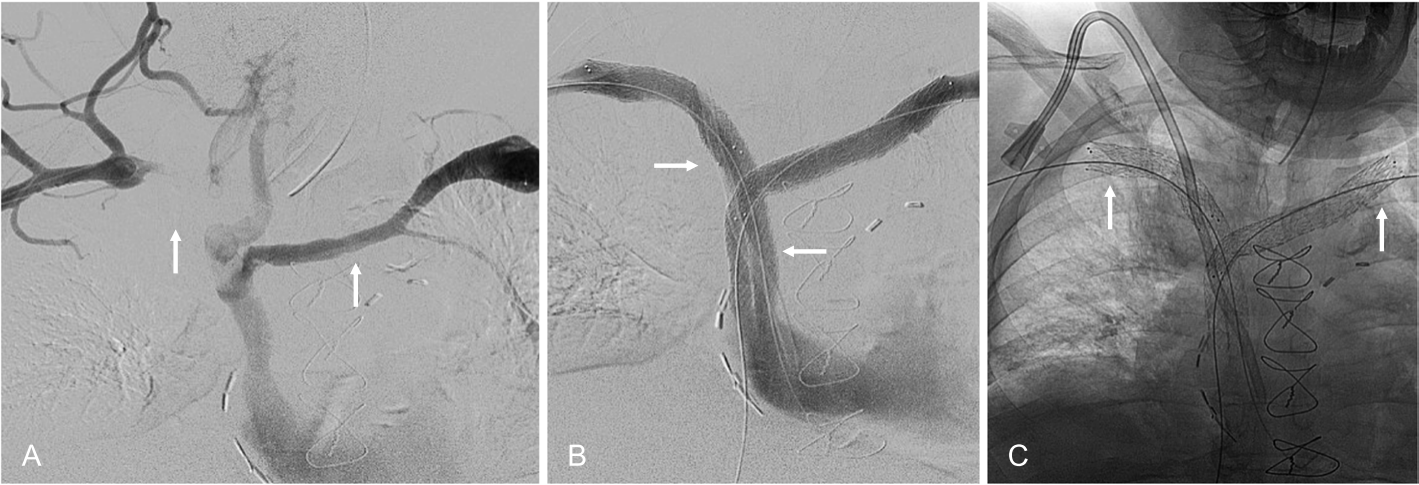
65-year-old male with end-stage renal disease with superior vena cava syndrome* (A) Bilateral upper extremity venography demonstrating bilateral brachiocephalocaval vein occlusion (arrows) (B) Bilateral upper extremity venography, after bilateral brachiocephalocaval stent reconstruction using bilateral 11-mm VBX balloon expandable endoprostheses (centrally) and 14-mm Abre Venous Stents (peripherally) (arrows), showing brisk in-line from both upper extremities to the right atrium (C) Radiograph demonstrating the 14-mm Abre stents in the thoracic outlets (arrow) without deformation or fracture
